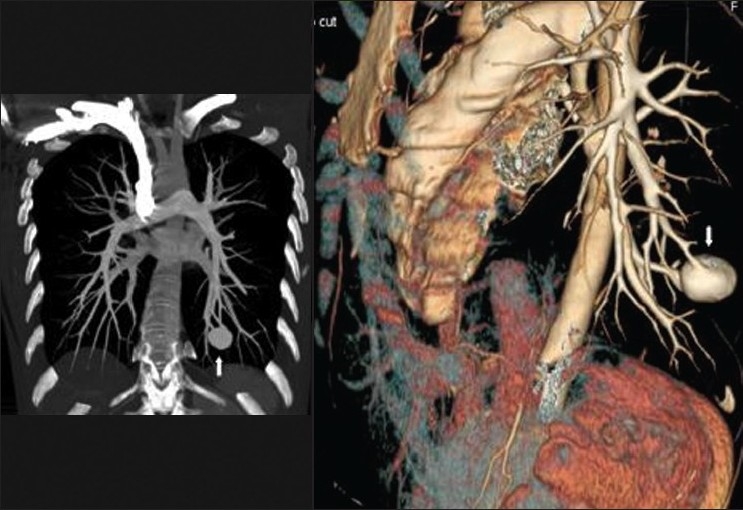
It is important that larger right to left shunts through pulmonary arteriovenous malformations are excluded by cross-sectional imaging when testing for HPS and when consideration is being given for hepatic radioembolization with Yttrium-90 glass microspheres. These coronal and sagittal reconstructions of contrast enhanced CT show a large arteriovenous malformation at the left lung base
